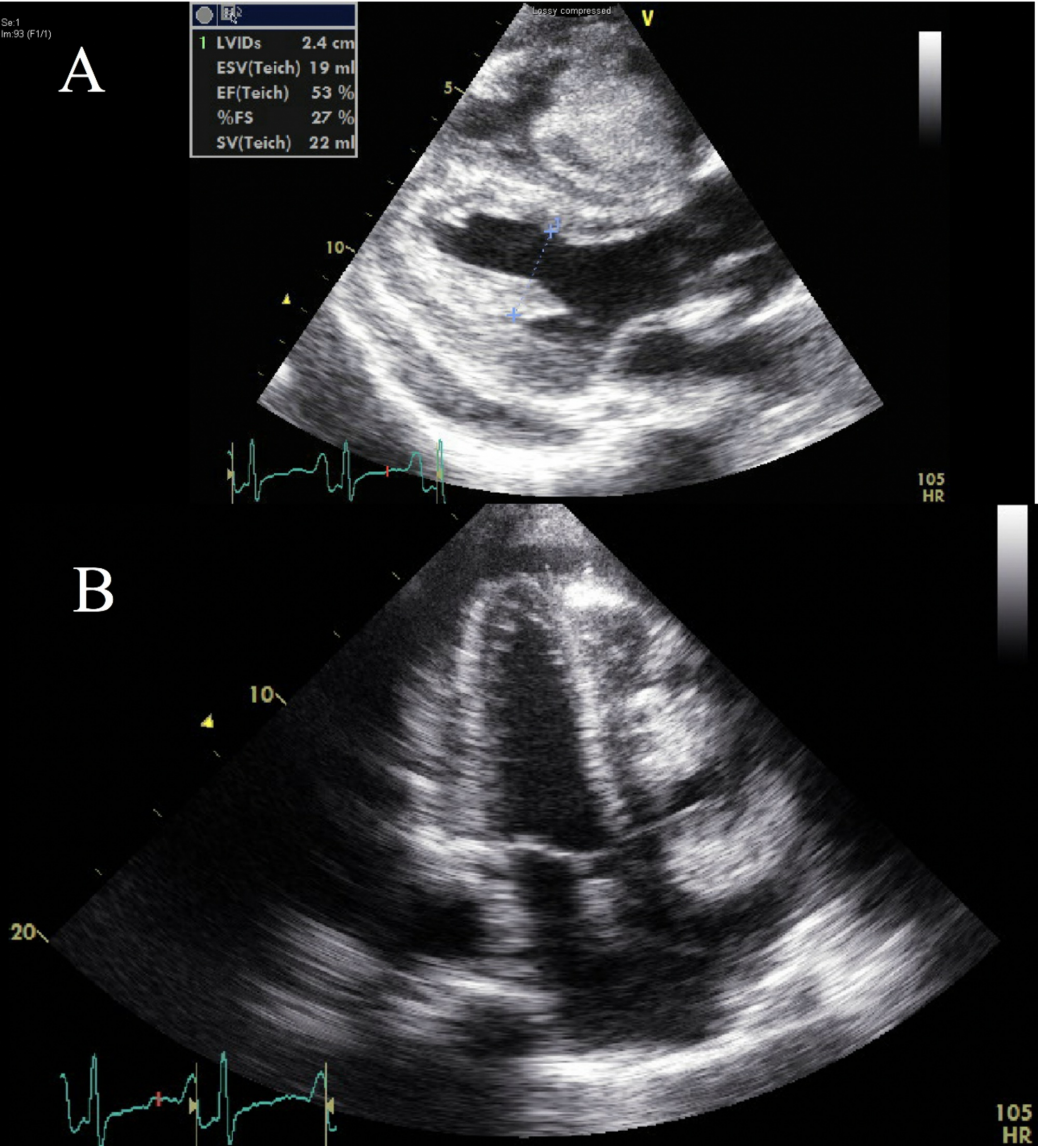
Follow-up Echocardiogram Imaging A) Parasternal long axis and B) apical four-chamber echocardiography views at a six-week interval showing a large mass in the right ventricle.
